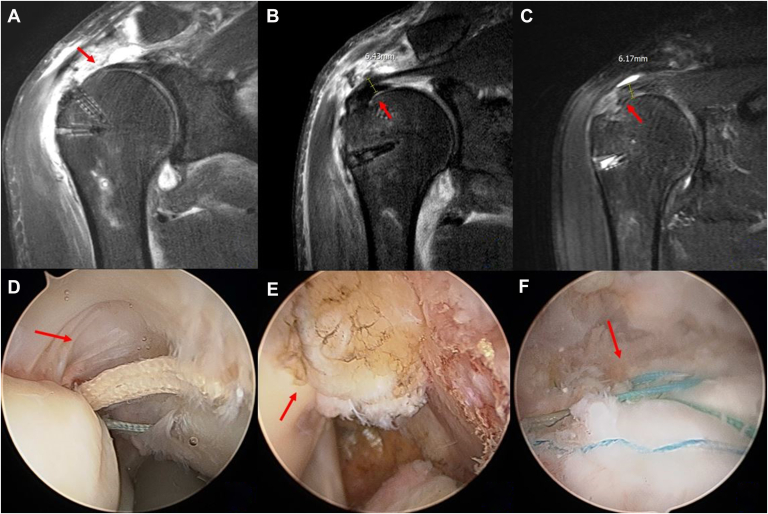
Tendon thickness and arthroscopic findings. MRI findings of the revision rotator cuff repair with biceps tendon augmentation without tear completion. (A) A coronal T2 fat-suppressed MRI scan of the supraspinatus tendon showed ill-defined contour and irregular intermediate T2 signal intensity change (*arrow*) in which was compatible with a PASR (partial articular-side supraspinatus retear) lesion. (B) A revision rotator cuff repair with biceps tendon augmentation (*arrow*) without tear completion for PASR lesions was performed. (C) 6 months postoperatively, follow-up MRI demonstrated a good incorporation of the augmented biceps tendon (*arrow*) into the native tendon. (D) Arthroscopic finding showed high-grade PASR lesion (*arrow*) and the bursal side of the supraspinatus tendon remained intact. (E) The augmented biceps tendon remained well incorporated into the rotator cuff footprint (*arrow*) in the glenohumeral joint. (F) In the subacromail space, transtendon suture bridge rotator cuff repair (*arrow*) was performed. *MRI*, magnetic resonance imaging.
